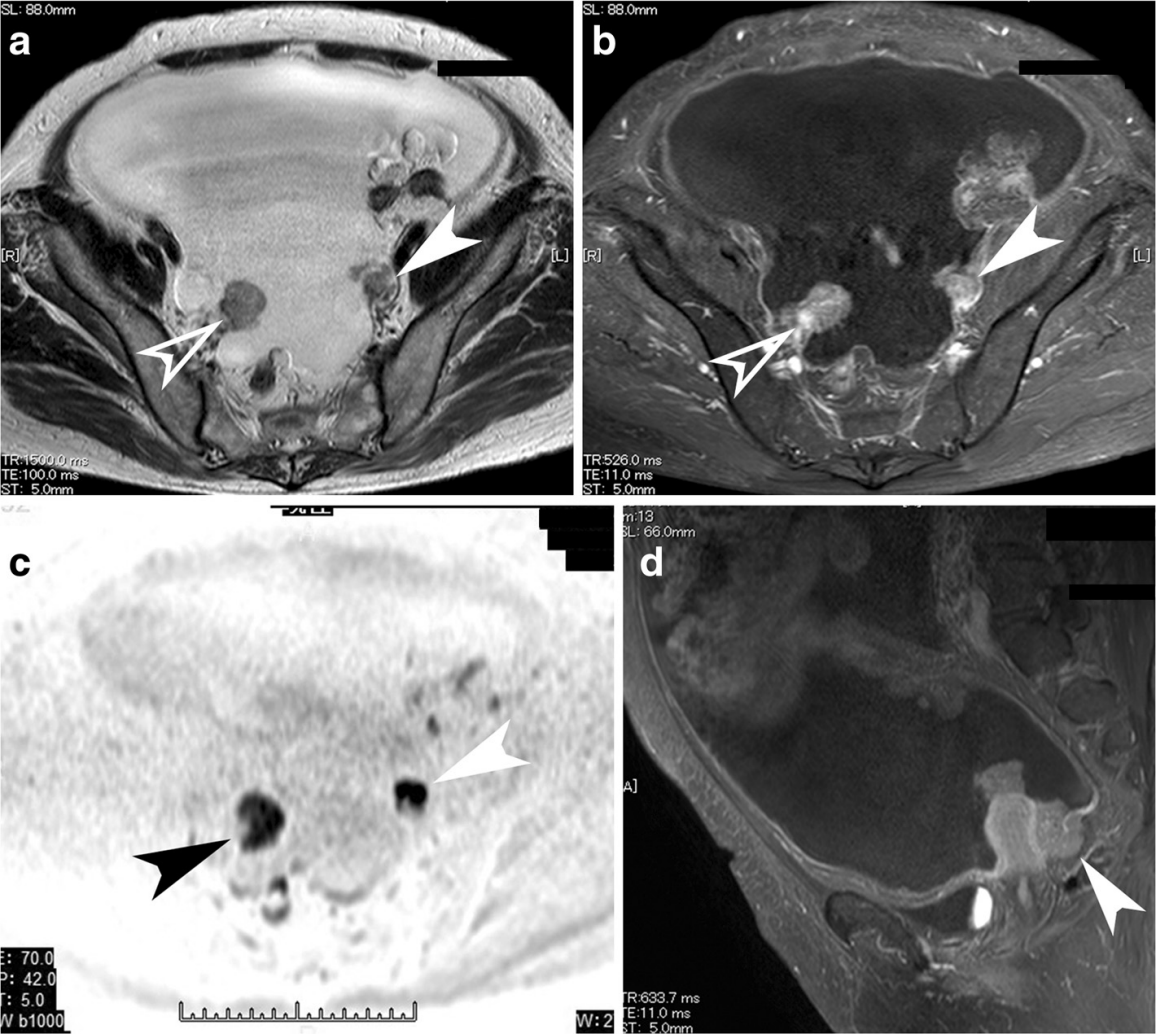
A case with serous carcinoma. Small almost entirely solid small masses are replacing the bilateral ovaries (arrows, a: axial T2-weighted image, b: axial contrast-enhanced and fat-saturated T1-weighted image). Both masses show strong restricted diffusion on diffusion-weighted image (c, arrowheads). Note the massive ascites and thickened parietal peritoneum with a mass in the cul-de-sac (arrowhead) on sagittal and contrast-enhanced and fat-saturated T1-weighted image (d), reflecting the extensive peritoneal dissemination
